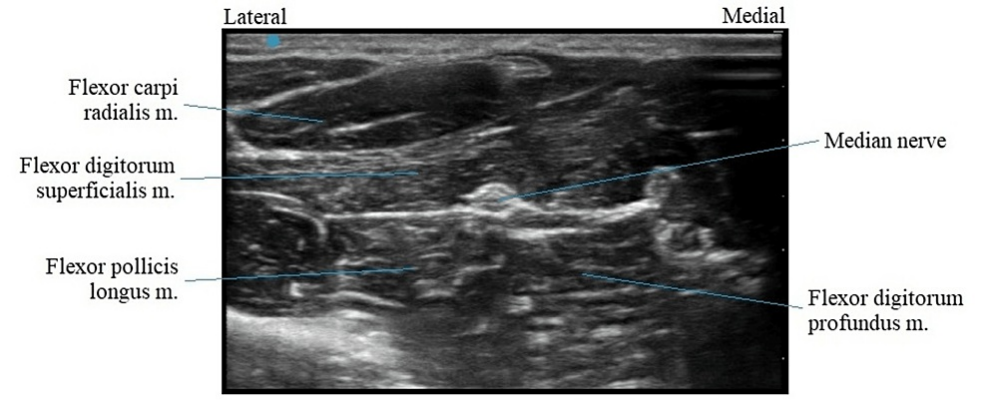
Ultrasonography of the median nerve m.: muscle
